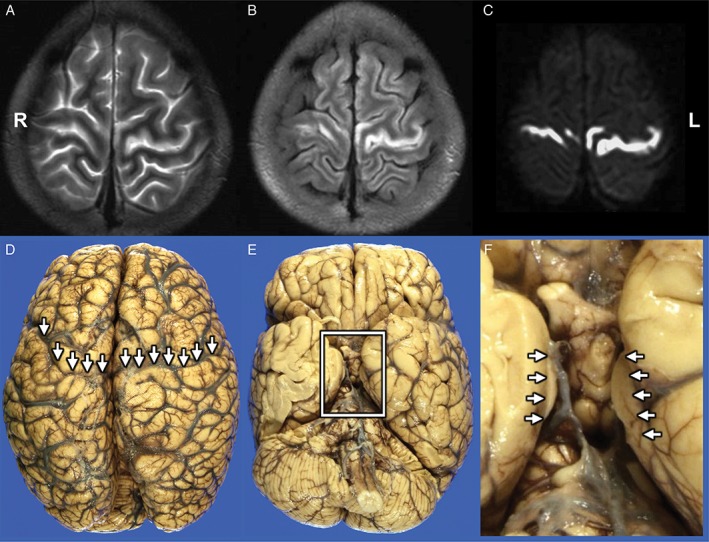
Brain MRI findings (A‐C) and gross appearance of the autopsied brain (D‐F). Hyperintenses areas of both the precentral gyrus are visualized on a T2WI (A), an FLAIR image (B), and a DWI (C). The brain shows massive edema (D, E). Arrows indicate both the precentral gyri (D). Box in (E) and arrows in (F) indicate bilateral uncal herniation.
